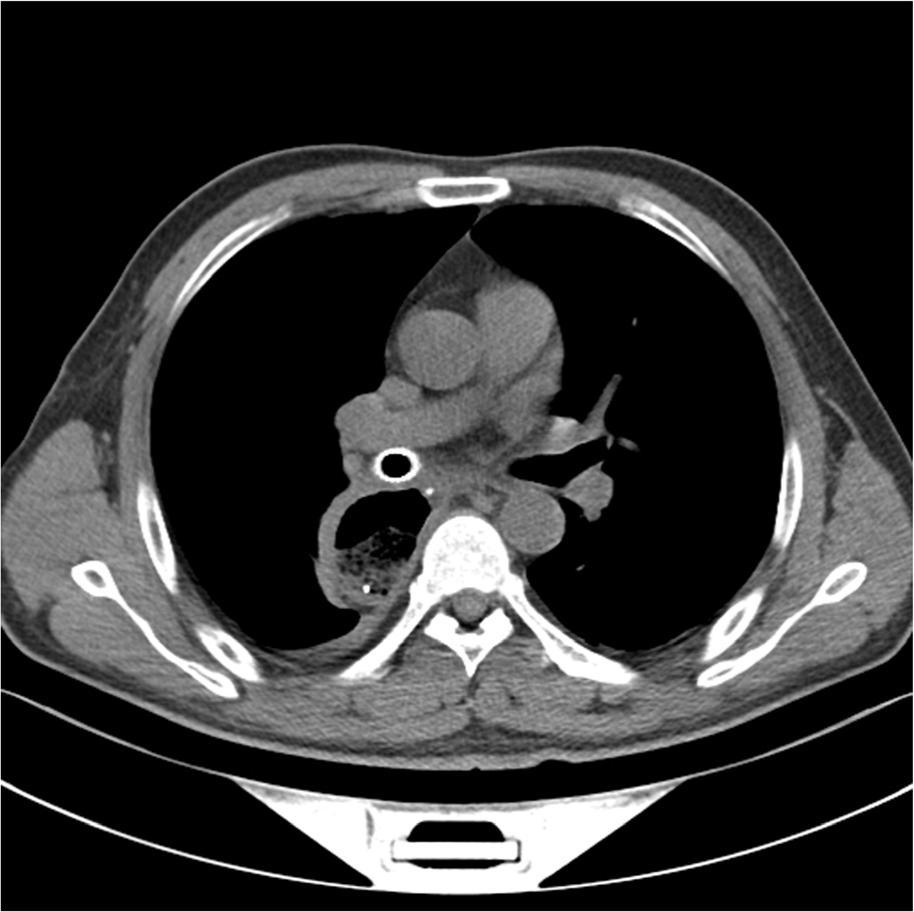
CT shows the fistula tract was cured
